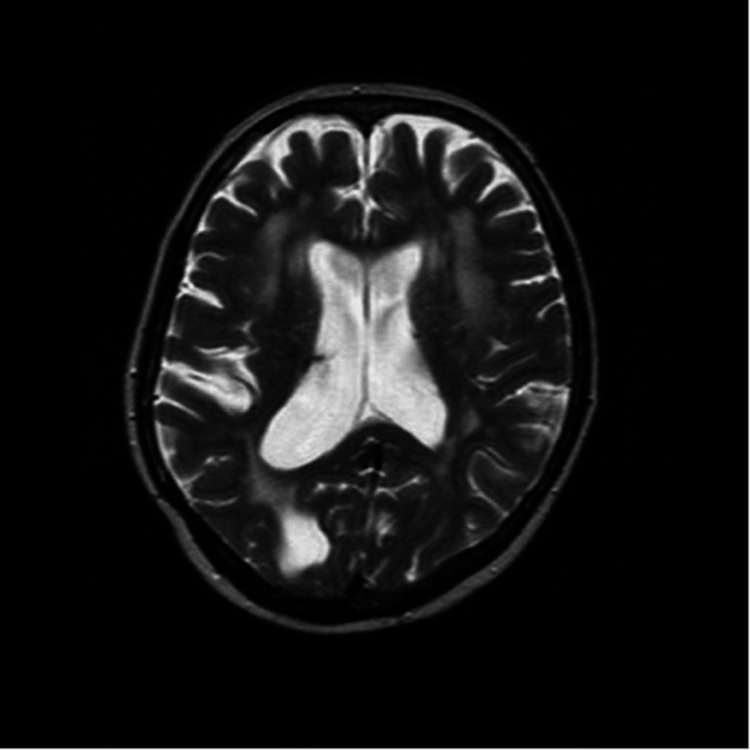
T2 weighted MRI showing ventriculomegaly and prominent sulci present in an ex-vacuo fashion, with extensive white matter disease throughout the supratentorial white matter related to previous glioblastoma treatment.
